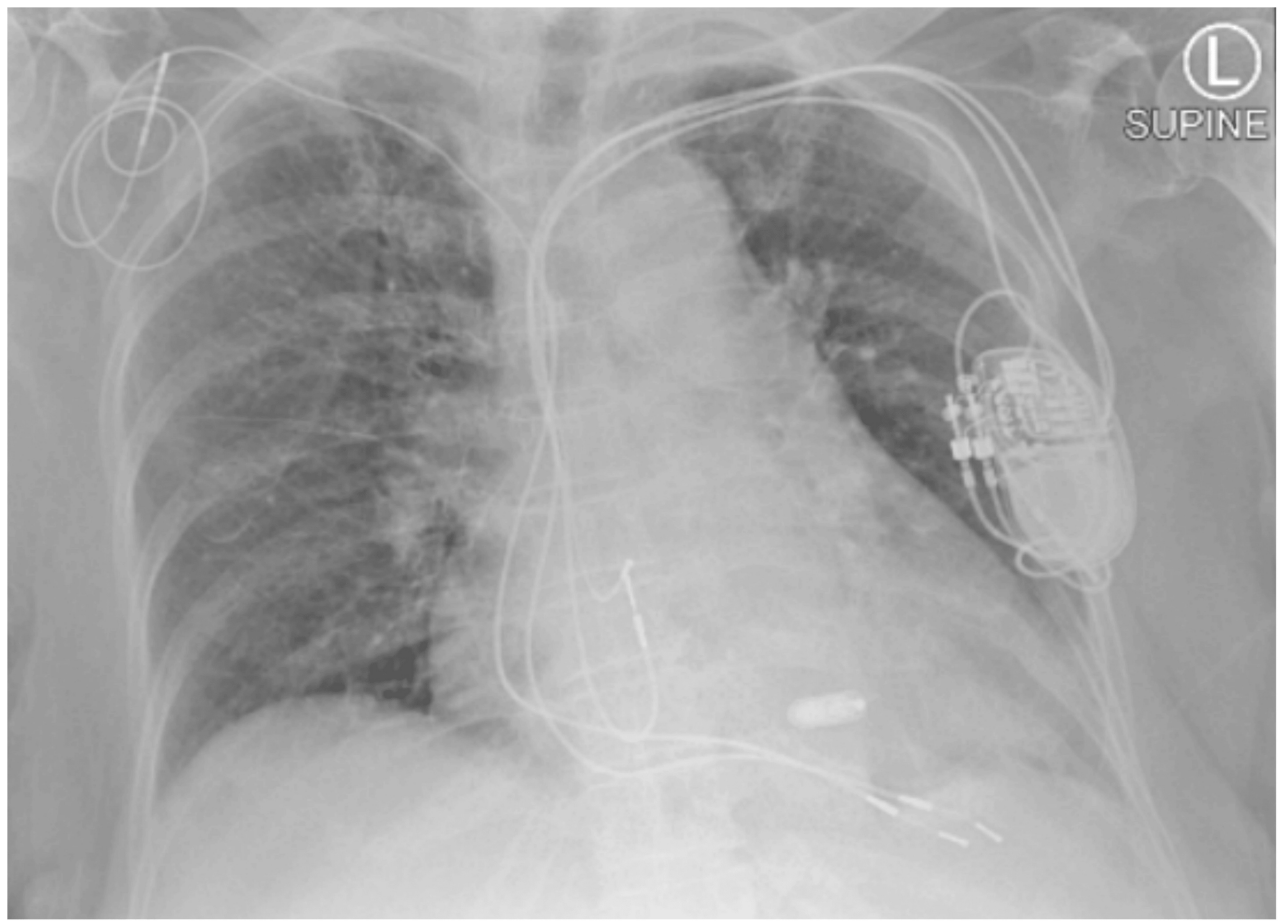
Chest X-ray after leadless pacemaker implantation Perform a chest X-ray to assess the positioning of both leadless and traditional pacemaker systems. Confirm the placement of the leadless pacemaker by verifying its location in the appropriate septal region and ensuring there is no dislodgement of transvenous leads after implantation.
